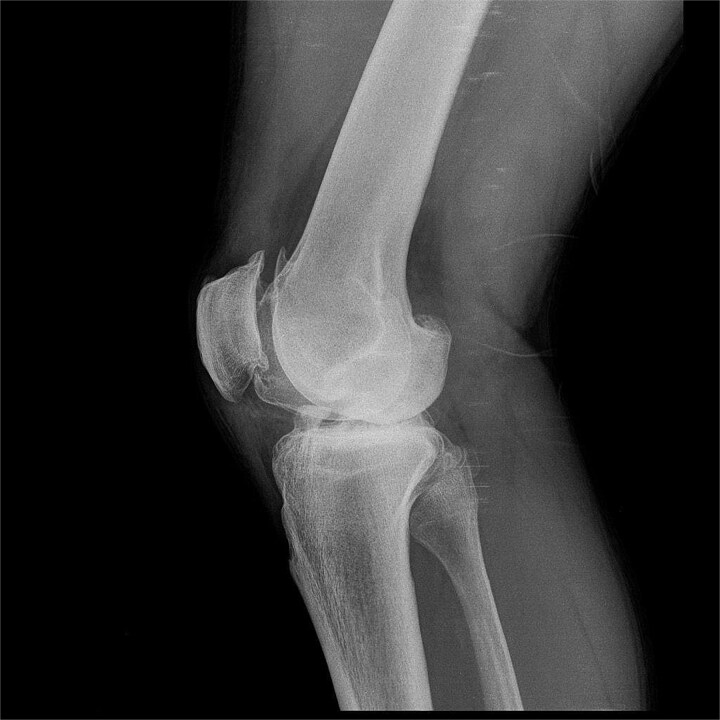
Lateral X-ray of the right knee showing marked joint involvement.
